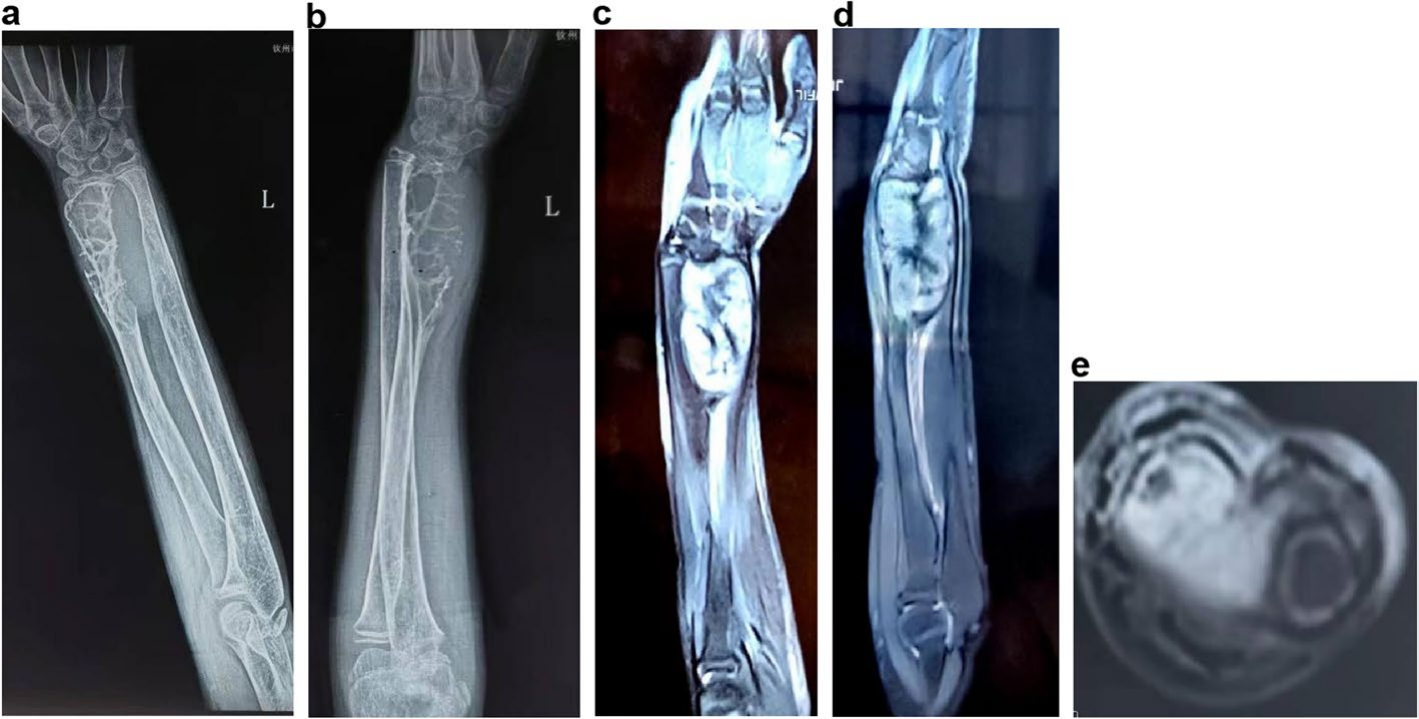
a Anteroposterior (AP) and b lateral radiographs reveal extensive osteolytic lesions in the distal radius; internal pseudo-trabeculations are observed. Magnetic resonance imaging (MRI) shows a large mass with a clear and smooth rim invading the radial metaphysis and carpal articular surface. c Coronal view. d Lateral view. e AP views
